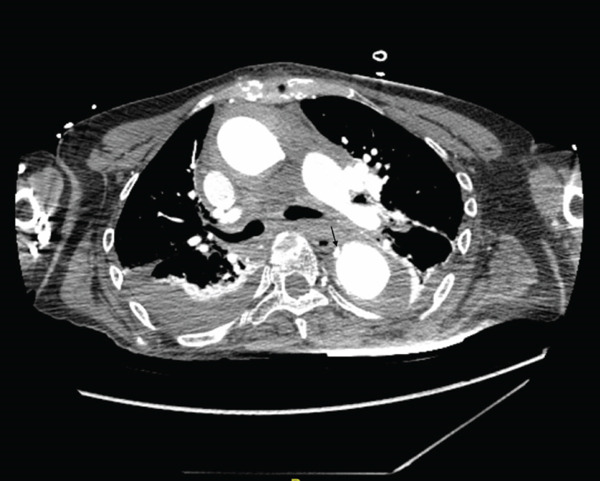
CT findings showing focal small outpouching at the left lateral wall of the ascending thoracic aorta, aortic intramural hematoma at the diaphragmatic hiatus and infrarenal abdominal aorta, hemopericardium measuring up to 15 mm in thickness and small layering bilateral pleural effusions.
